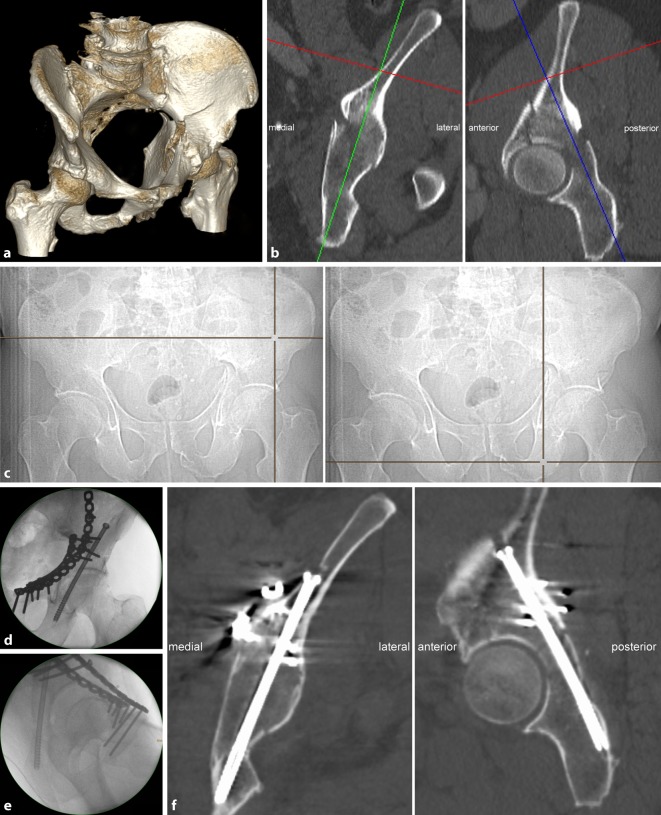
a Posterior column screw—fracture type. A 67-year-old man who sustained a bicycle accident with a direct impact on the left hip. The CT scan shows an anterior column with posterior hemitransverse fractures (ACPHF) with a multifragmentary fracture of the anterior column. The quadrilateral plate is internally rotated and in osseous continuity with the posterior column. The posterior hemitransverse fracture is simple as shown in b. b Preoperative planning—posterior column screw trajectory. The medial angle of the posterior column screw trajectory is 16° to the sagittal plane (*left*) and the posterior angle is 24° to the coronal plane (*right*). The maximum screw length is 123 mm. c Preoperative planning—estimation of the starting point and the endpoint. The starting point and the end point are determined using the LiveSync feature as described in Fig. 3. d Intraoperative application—fluoroscopic control in anterior–posterior (ap) view. The anterior column fracture was reduced via a modified Stoppa approach and stabilized with two 3.5 pelvic reconstruction plates. The posterior hemitransverse fracture was indirectly reduced by reducing the quadrilateral plate. The starting point for the posterior column screw was approached via a small incision at the iliac crest and submuscular preparation under fluoroscopic control in the ap view. The guide wire was advanced according to preoperative planning (b). It is advisable to switch to the lateral–oblique view when approaching the hip joint. After predrilling, a cannulated 6.5 mm screw with a 32 mm thread and a length of 115 mm was inserted. The screw length must not be longer than the maximum length determined in preoperative planning, but may be shorter provided that the screw thread completely passes the fracture line. The fluoroscopic control shows a screw trajectory as preoperatively planned (c). e Intraoperative application—fluoroscopic control in lateral–oblique view. The left hip joint is located next to the radiation source which results in a “larger” left hip joint. Additionally, the radiation source is tilted 15° upwards resulting in a more posterior projection of the left hip. The lateral–oblique view shows that the screw does not penetrate the hip joint and does not perforate the cortical bone of the posterior column and the sciatic tuber. f Postoperative CT control. The correct screw trajectory is confirmed in the postoperative CT scan. The posterior hemitransverse fracture is anatomically reduced
